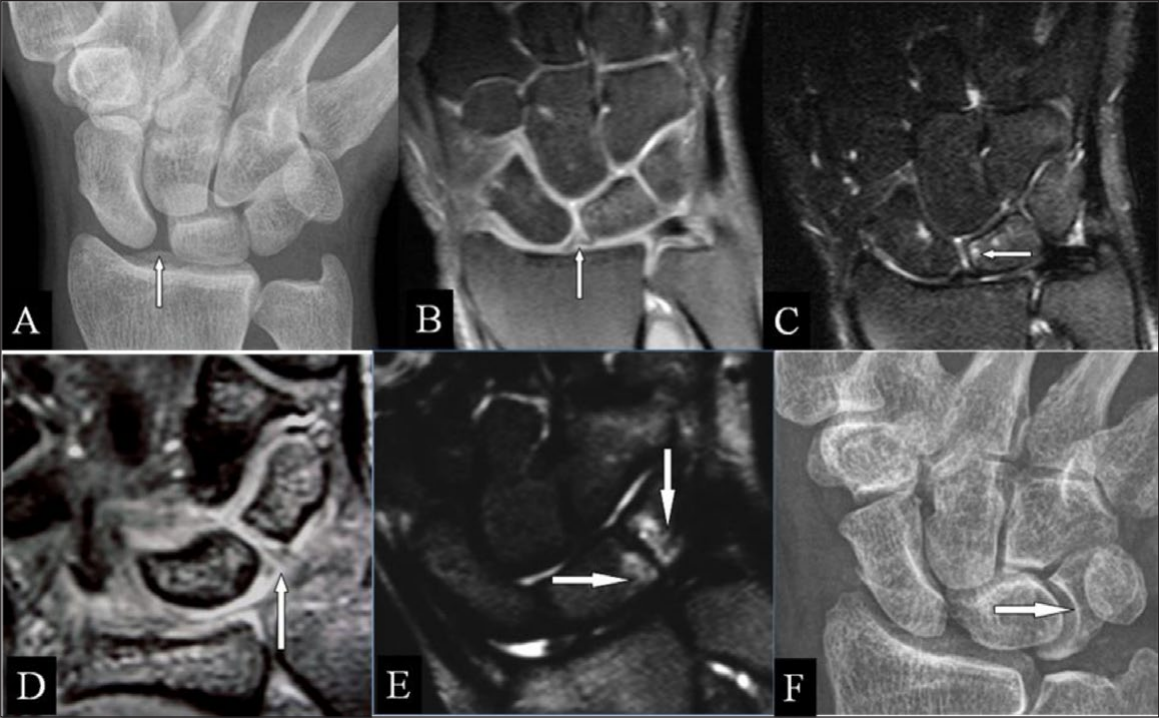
Scapholunar and lunotriquetral abutment. (A) PA plain radiograph; (B) Coronal SE PD-WI FS; (C) Coronal SE T2-WI FS; (D) Coronal 2D-GRE; (E) Coronal SE T2-WI FS; and (F) PA plain radiograph. (A) Widening of the scapholunate joint space on a Schneck I view. (B) Tear of the scapholunate ligament. (C) Juxta-articular subchondral band shaped marrow oedema. (D) Tear of the lunotriquetral ligament. (E) Juxta-articular band shaped kissing marrow oedema. (F) Massive deformation at the triquetral bone (other patient).
